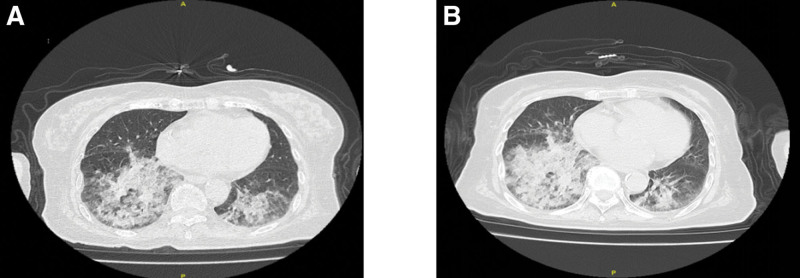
Chest computed tomography changes in the lungs of patients undergoing 1 cycle of treatment. (A) May 04, 2024. (B) May 10, 2024.
